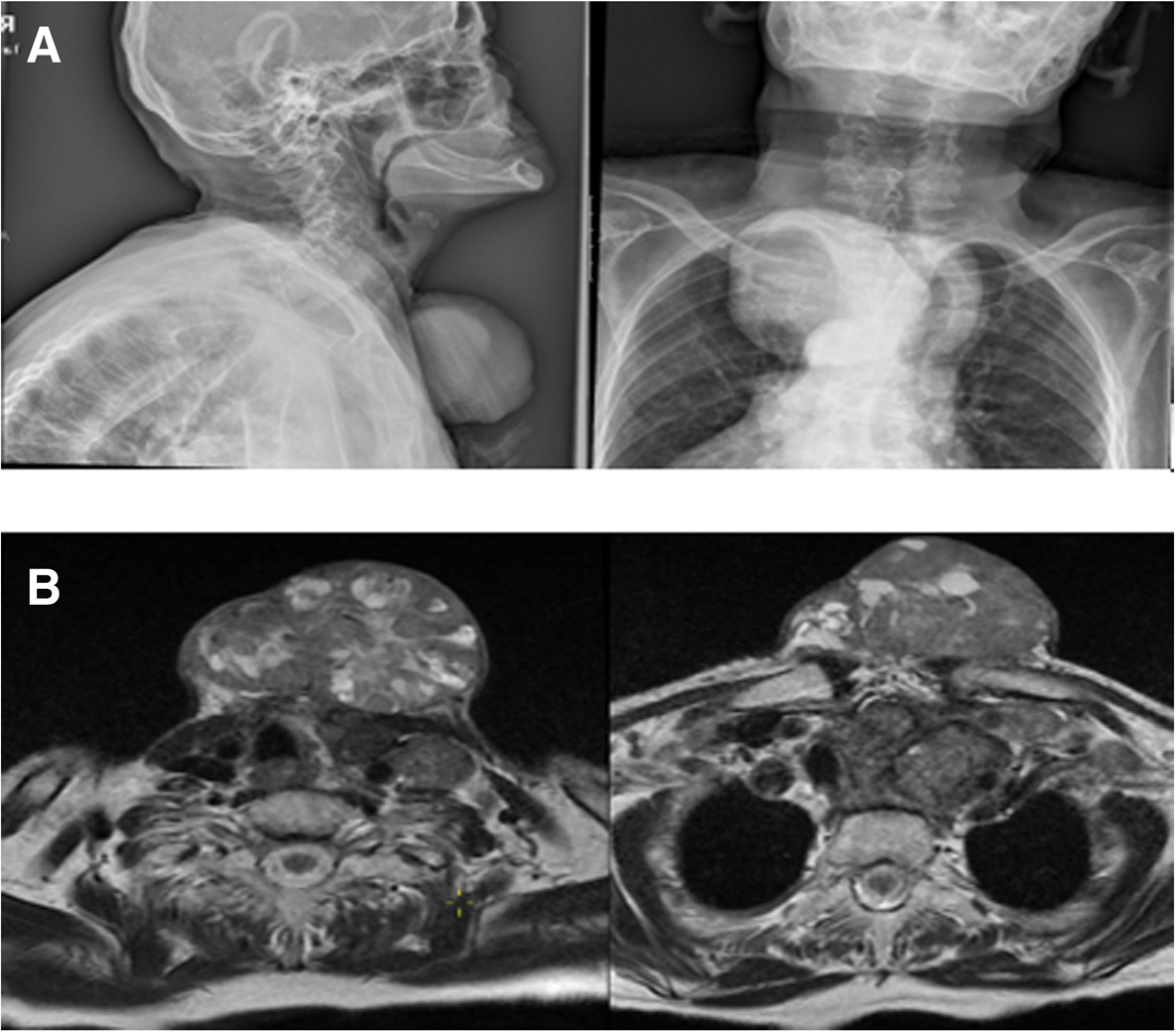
Screening images of the cervical mass, displayed by lateral anteroposterior X-ray (a) and cervical magnetic resonance imaging (b)
